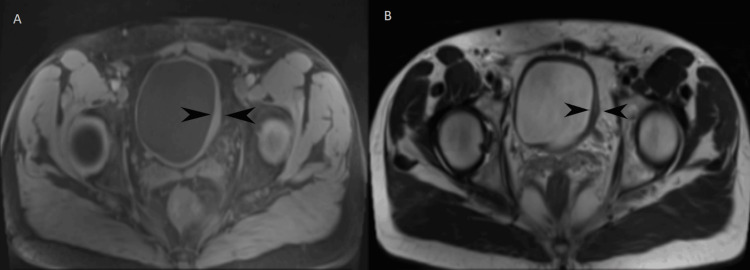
MRI of the lower abdomen one month post-surgery. A: T1-weighted imaging; B: T2-weighted imaging. The only finding was thickening of the bladder wall, likely due to postoperative alterations.
